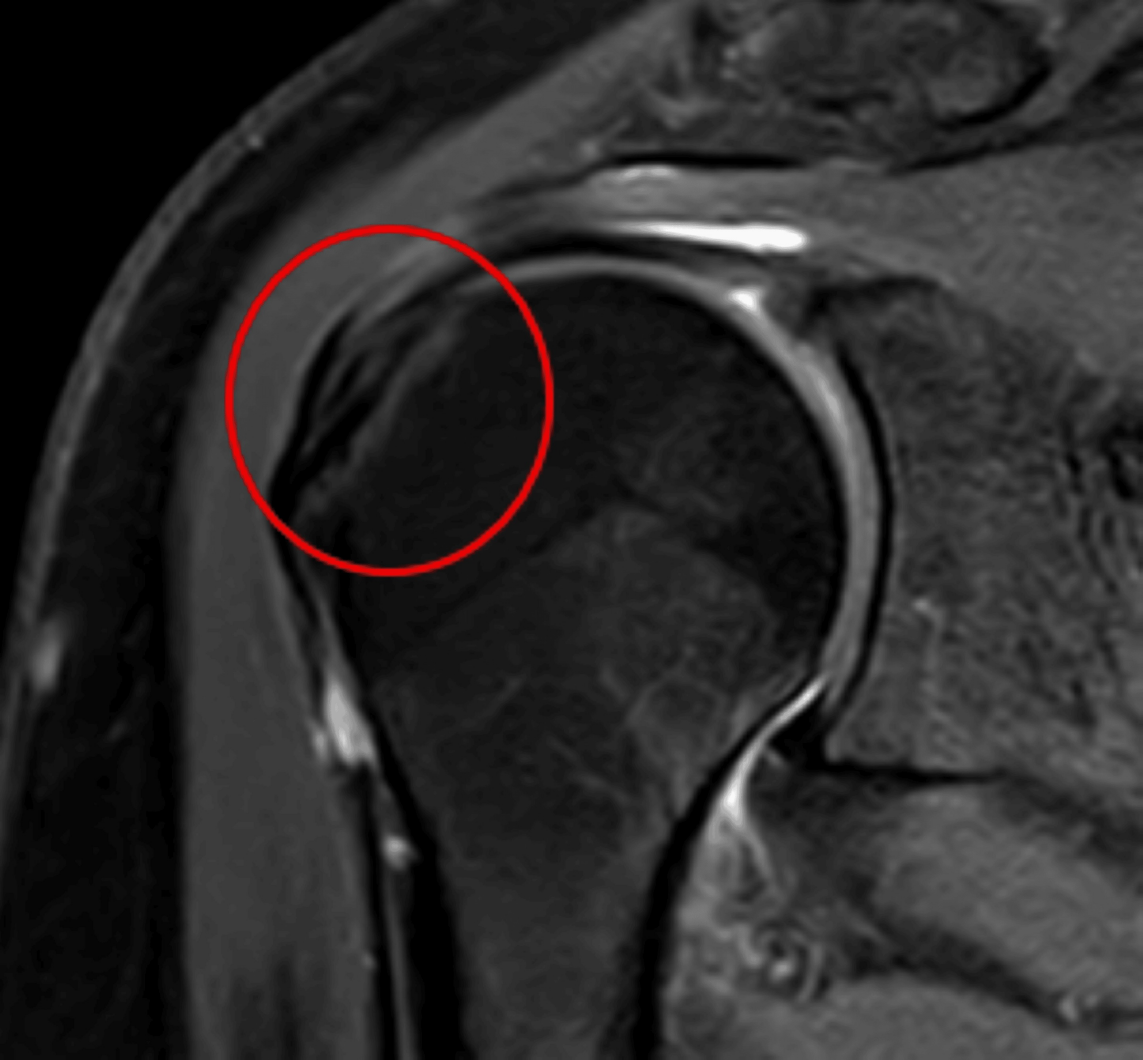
The MRI of the left shoulder joint signifies a partial thickness tear in the rotator cuff muscle (circle)
